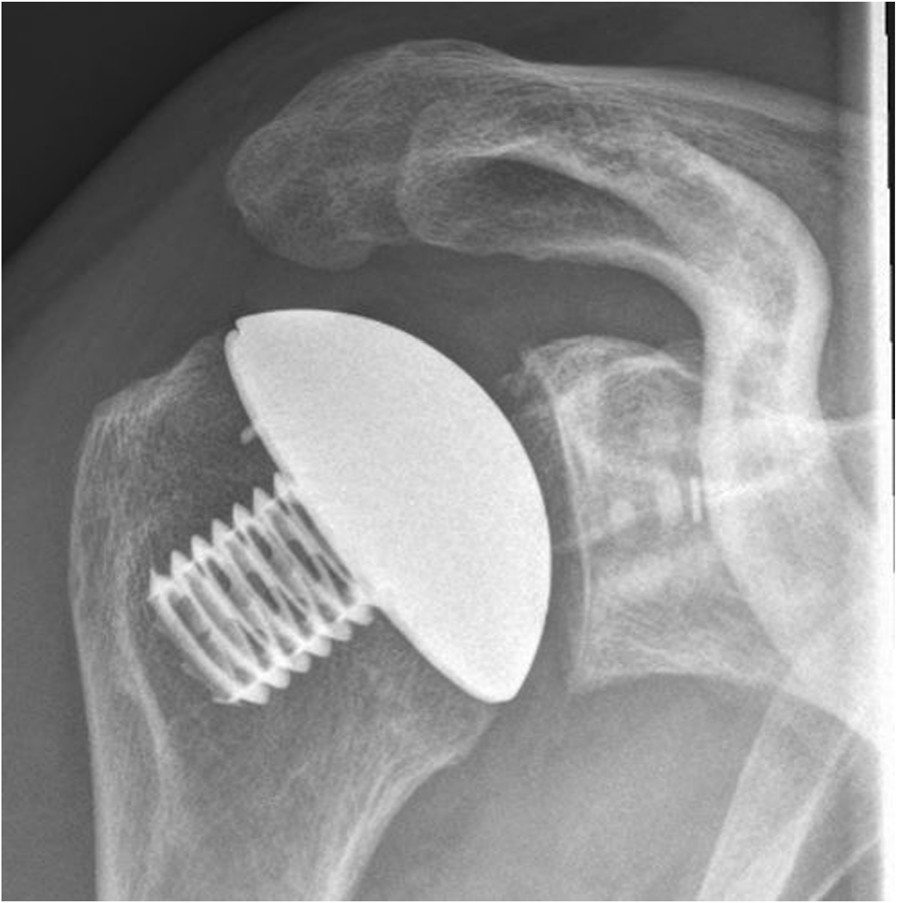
Radiograph shoulder in true-AP: eclipse without calcar resorption
